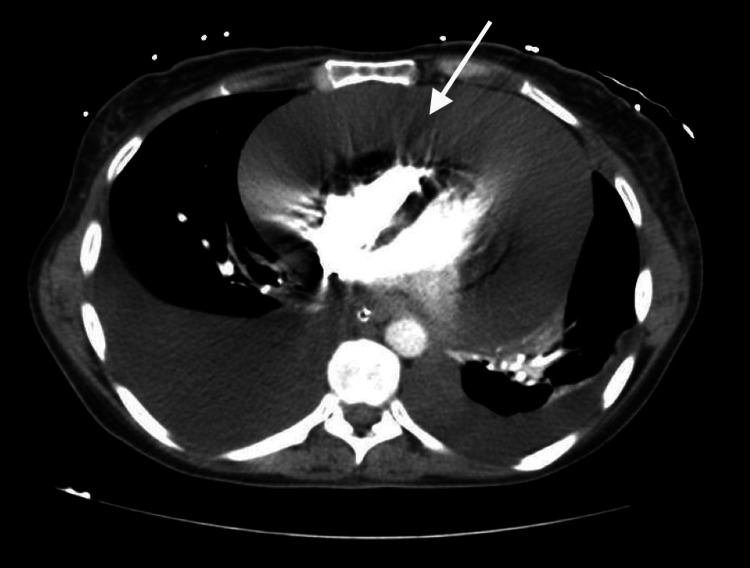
Pleural and pericardial effusion (arrow) on CT thorax
